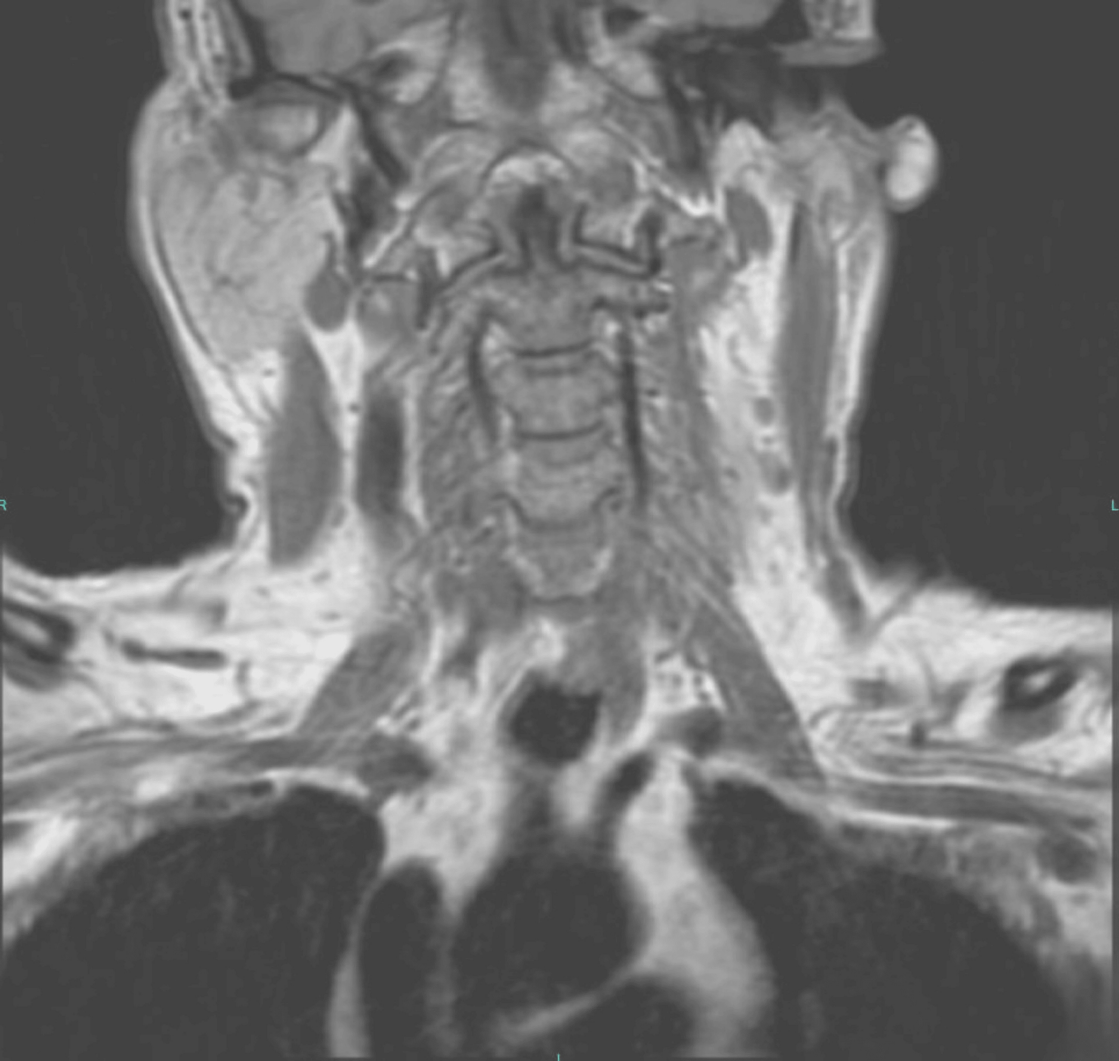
Case B: MRI of brachial plexus. There is no intrinsic mass or extrinsic compression of the left brachial plexus.
